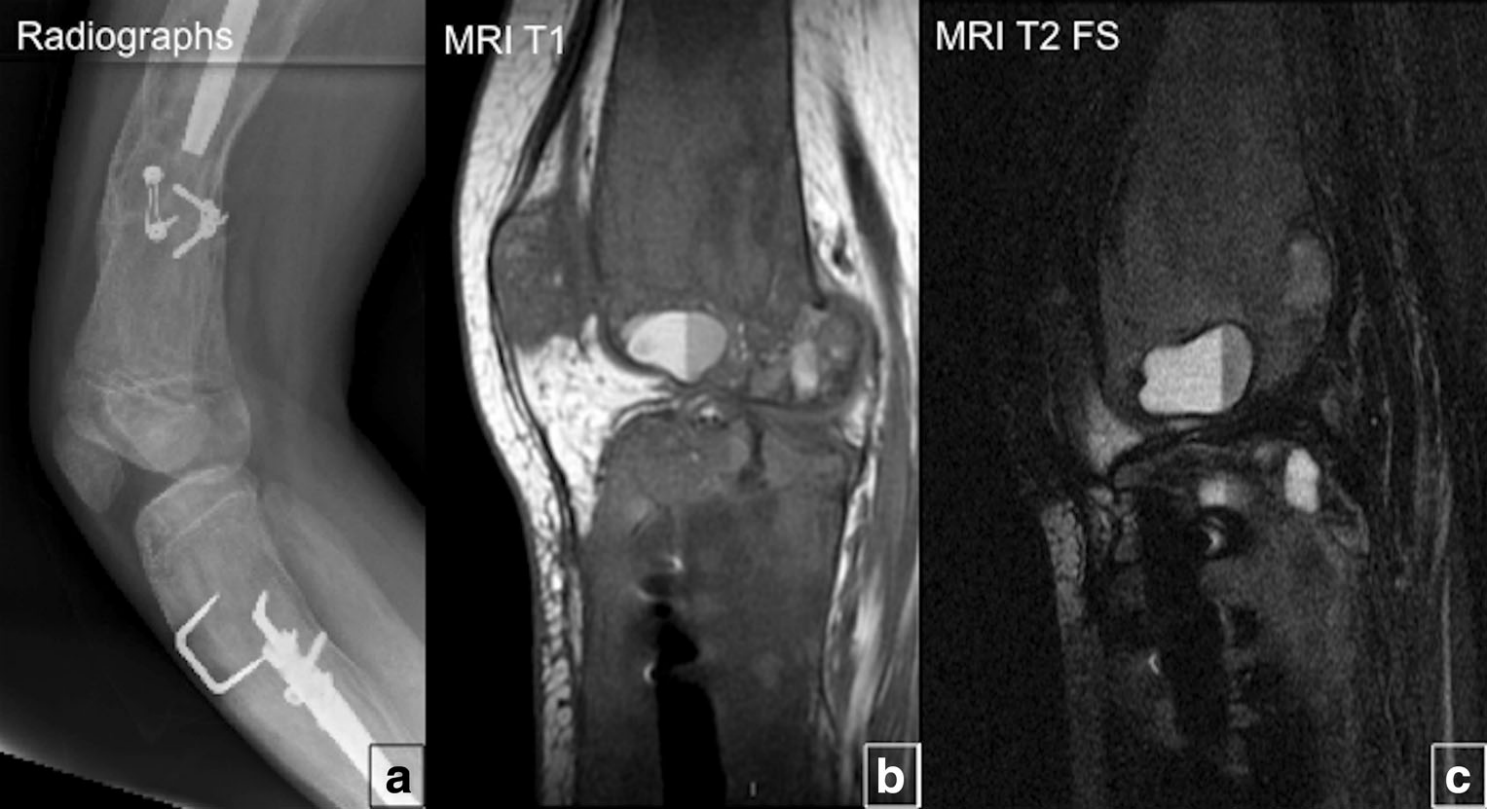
Benign fibrous dysplasia (FD) bone matrix transformation to aneurysmal bone cysts (ABCs). a Radiographs of the distal femur and the proximal fibula show heterogeneous FD lesions with surgical hardware. T1-weighted MRI (b) and T2 fat-suppressed images MRI (c) demonstrate ABCs
